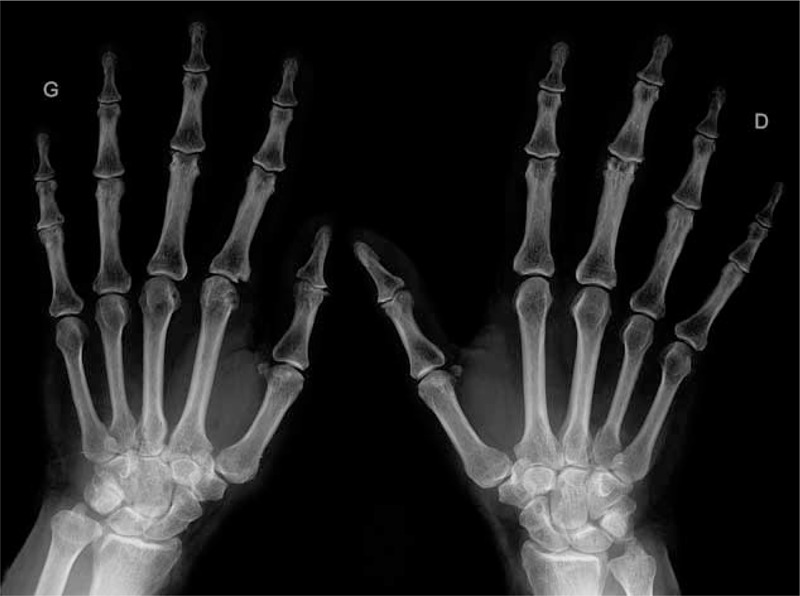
Representative hand radiographs in ASS patients with ACPA. ACPA = anticitrullinated peptide/protein antibody, ASS = antisynthetase syndrome.
